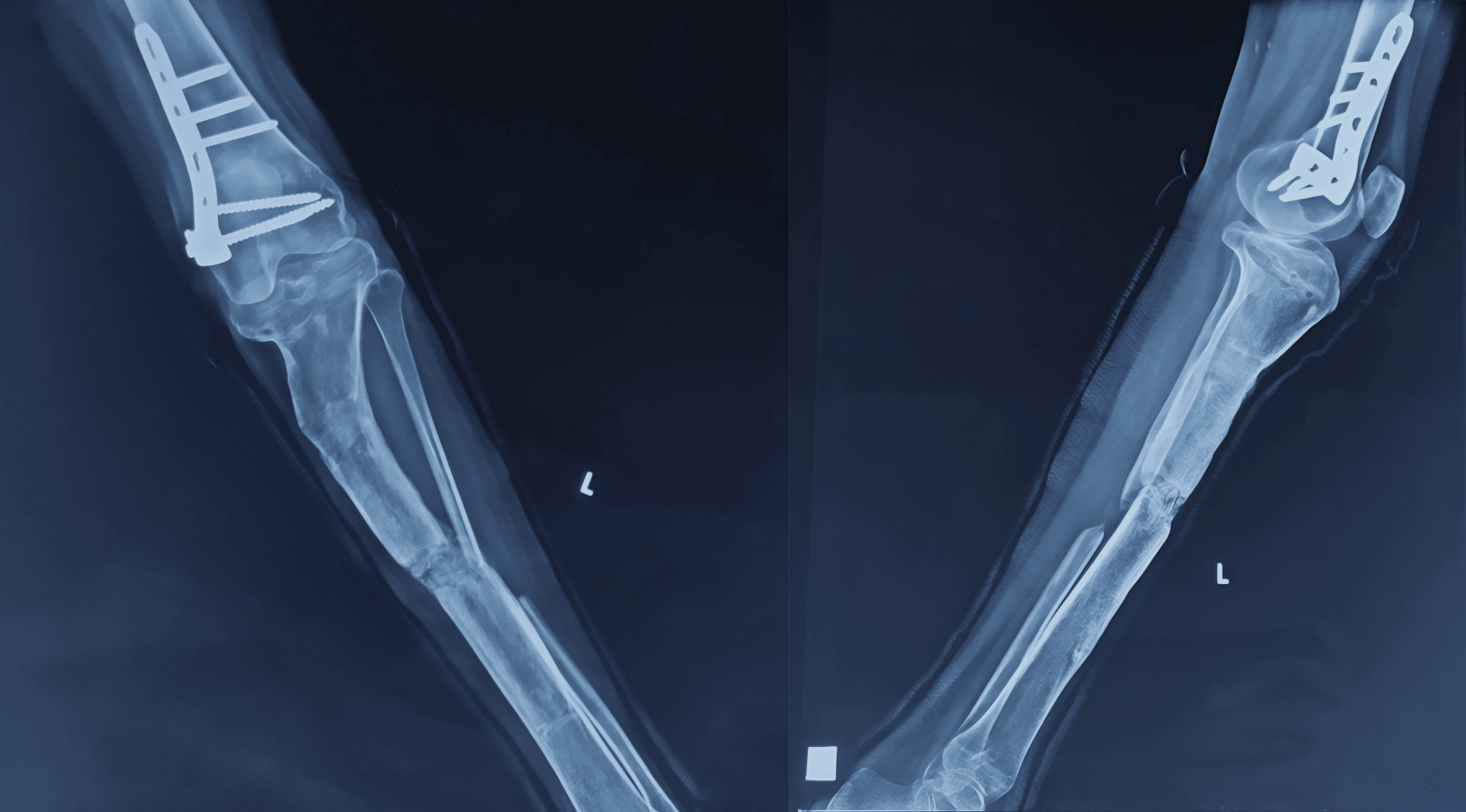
Two-year follow-up postoperative X-ray anteroposterior and lateral views showing good union in the tibia with a significant correction of the deformity.
